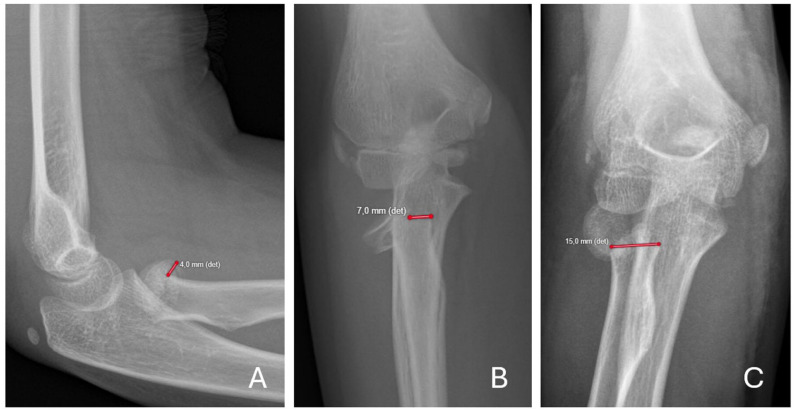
Maximal displacement was measured on both AP and lateral radiographs, and the greater value was recorded. Examples: (a) Judet type II fracture with 4 mm maximal displacement on the lateral view; (b) Judet type III fracture with 7 mm maximal displacement on the AP view; and (c) Judet type IV fracture with 15 mm displacement on the AP view. det: detector; AP: Anteroposterior; distance was measured relative to the detector positioned beneath the arm.
